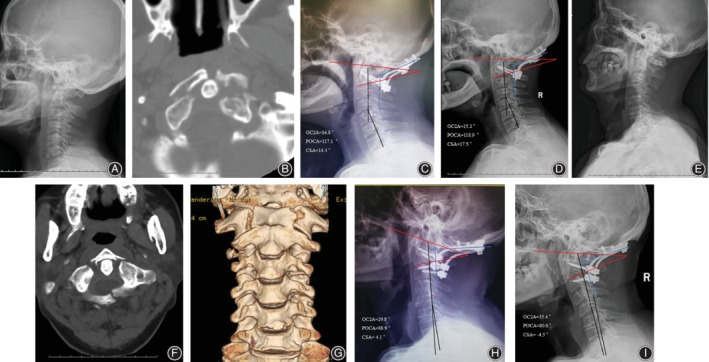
A 64 year old female with atlas fracture and atlantoaxial dislocation (A, B), who was established in an ideal angle of OC2A and POCA (both within 95% CI of the healthy population) during OCF (C). Cervical lateral radiograph showed a fairly good curvature of the lower cervical spine at 18 months follow‐up (D). On the contrary, a 62 year old male with Jefferson fracture (E, F, G), OC2A and POCA of the patient were established in a non‐ideal angle in OCF (H). The lower cervical spine of the patient had a kyphosis due to loss of the cervical curvature at 12 months after OCF (I).
